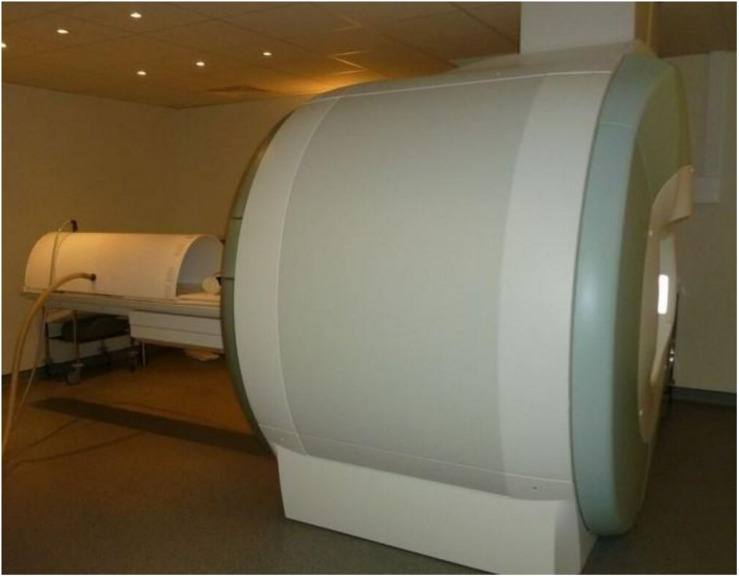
LBNP chamber, made with wood reinforced with plastic resin, especially designed for MRI scanner (Polyform^®^, La Mézière, France). Chamber was sealed with a neoprene suit. Negative pressure was maintained by air compressor (Mil’s, Lyon, France) and controlled by a manometer (ADMI, Noisy Le Grand, France) situated outside the MRI chamber.
